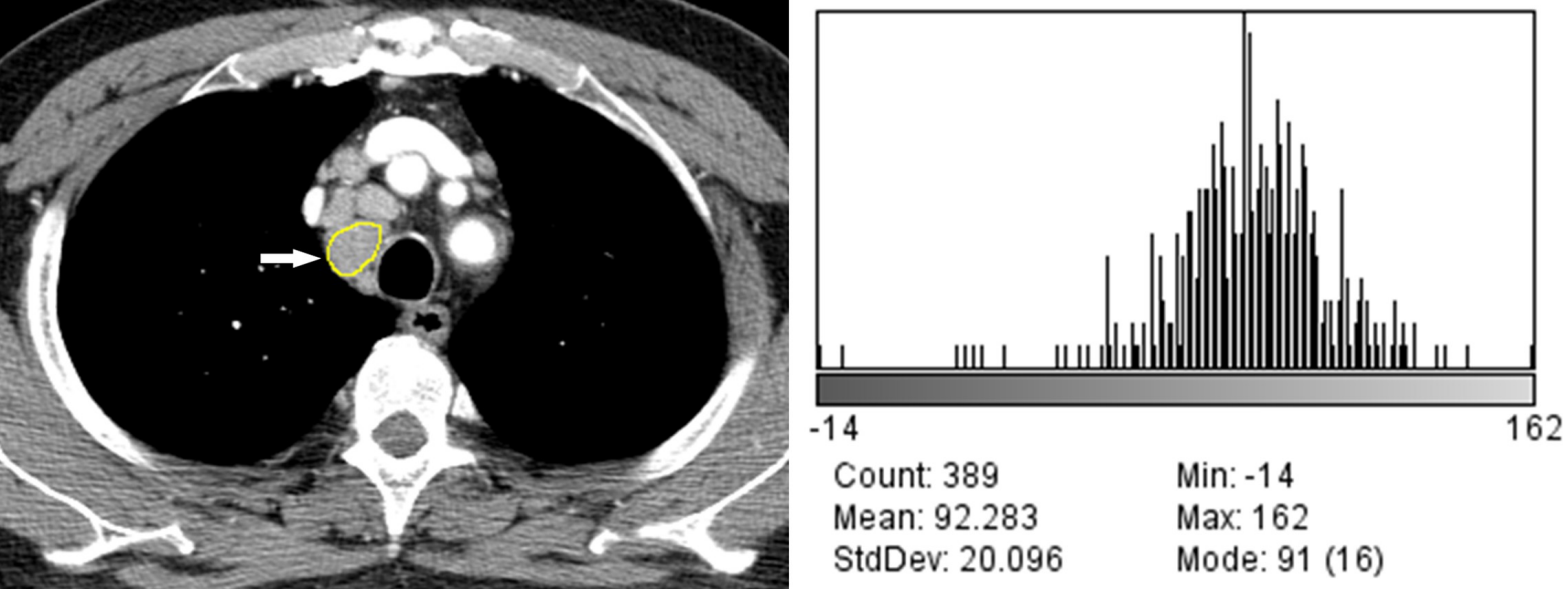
70-year-old man with sarcoidosis without lung involvement. (A) Mediastinal window image of contrast-enhanced chest CT scan shows a segmented right upper paratracheal lymph node (arrow). (B) Histogram plot shows the CT data profile of the segmented lymph node (skewness, -1.16; kurtosis, 5.75).
